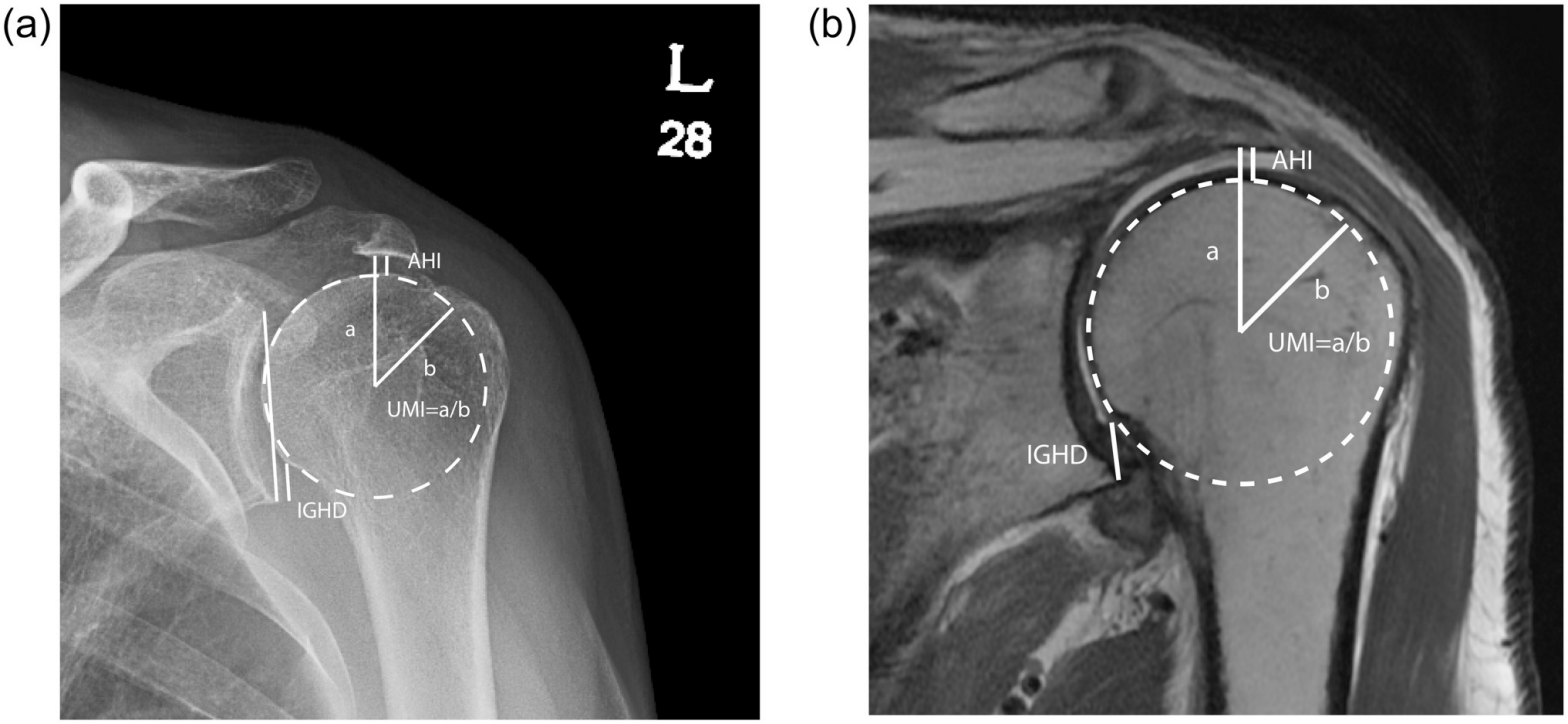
A-B Measurement of the superior humeral migration on anterior-posterior radiograph (A) and coronal magnetic resonance image (B). The acromiohumeral interval (AHI) was defined as the distance between the inferior acromion and the most superior aspect of the humeral head. The inferior glenohumeral distance (IGHD) was measured between the inferior glenoid margin and the inferior humeral head margin, which intersects the humeral head articular margin and the humeral neck. The upward migration index (UMI) was calculated as the distance between the lowest point on the acromion to the center of the humeral head (a), divided by the radius of the humeral head (b).
